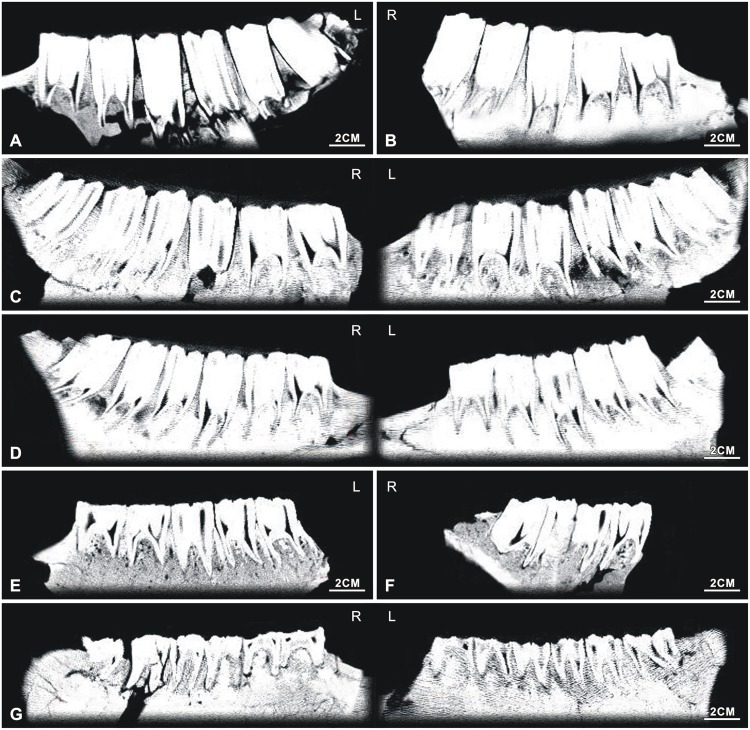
CT reconstruction images of the inlaid mandibular cheek teeth. (A) NWU1440.1; (B) NWU1439; (C) NWU1433; (D) NWU1430; (E) NWU1436; (F) NWU1444; (G) NWU1445.
